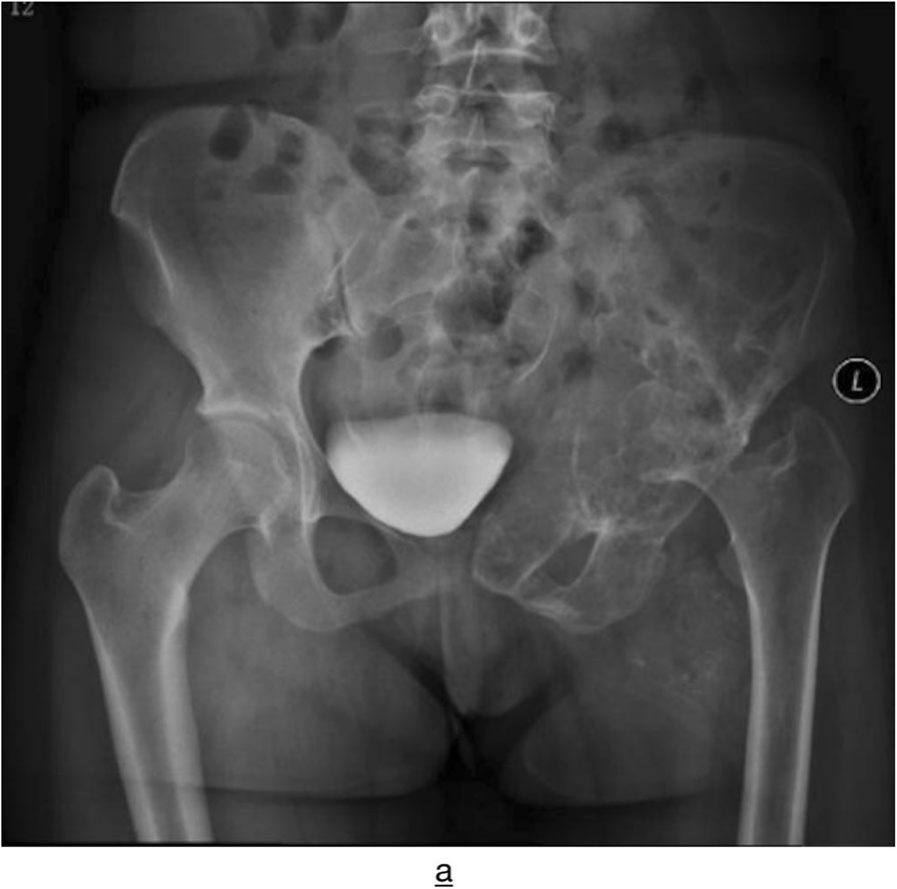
Radiograph showing osteolytic bone destruction of the left ilium, pubis, ischium, and femoral head, and subluxation of the left hip joint
